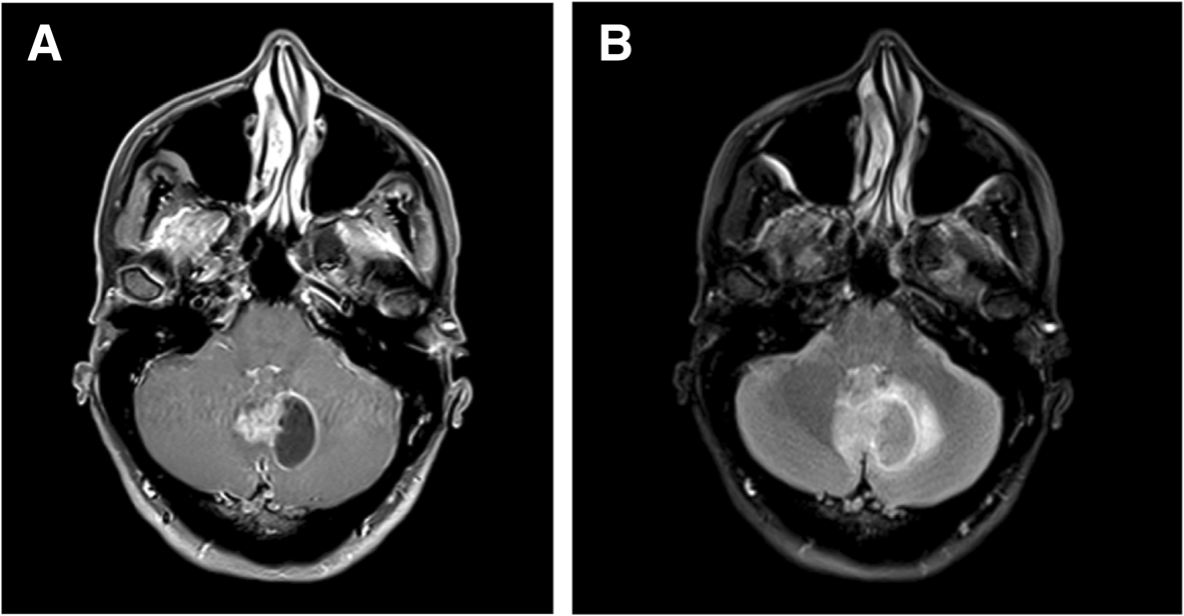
a) Axial T1 Post Gadolinium Fat Saturation. b) T2 FLAIR Fat Saturation
